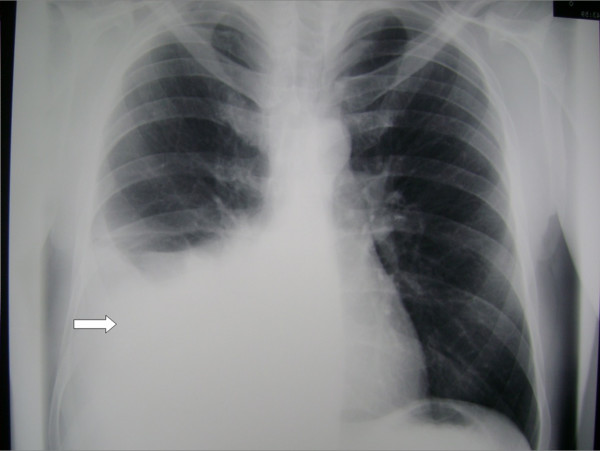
Chest retro-anterior radiograph showing a large right-sided pleural effusion without lung parenchymal disorders.
